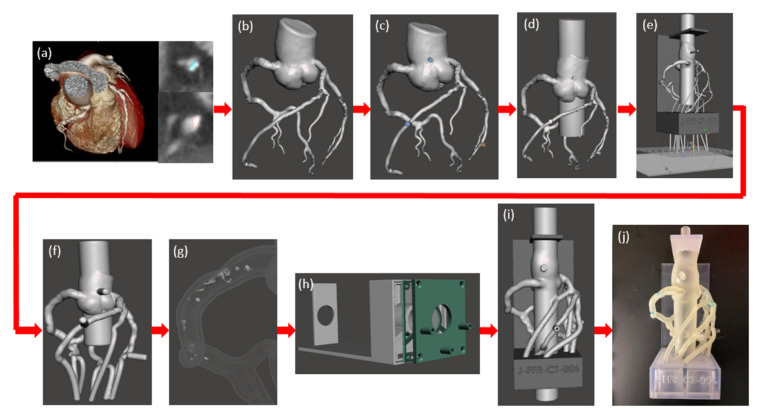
Model development process of 3D printed coronary artery model and experimental setup. (a) Coronary CT angiography scans of the heart tissue and the three main coronary arteries were imported for analysis. The coronary arteries were segmented separately from the calcification using thresholding and contouring methods. (b) A stereolithographic file was exported from volume data and imported into Autodesk Meshmixer and segmentation errors were removed. (c) Cylindrical meshes were appended to the aortic root and the diseased coronary artery for future pressure sensor connections. (d) The aortic root was extended at both the inlet and the outlet. (e) Vessel branches were extended through each of the three chambers and a plane cut was administered at the vessel outlets for parallel ends. (f) A 2mm wall was generated and the lumen was hollowed out. (g) The calcification was solidified and subtracted from the vasculature. (h) A three-chamber support structure was imported into Autodesk Meshmixer. (i) Then model and support structure are aligned and ready to be printed. (j) The model is 3D printed, cleaned, and ready to be attached to a flow loop. Reprinted with permission under open access from Sommer et al. [64].
